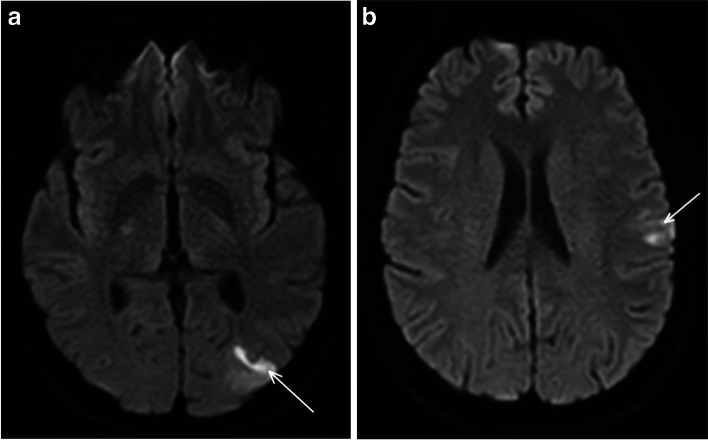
Concomitant acute ischemic lesions in the left MCA territory, in the parieto-occipital (a) and the frontal lobe (b); axial diffusion-weighted imaging DWI MRI
